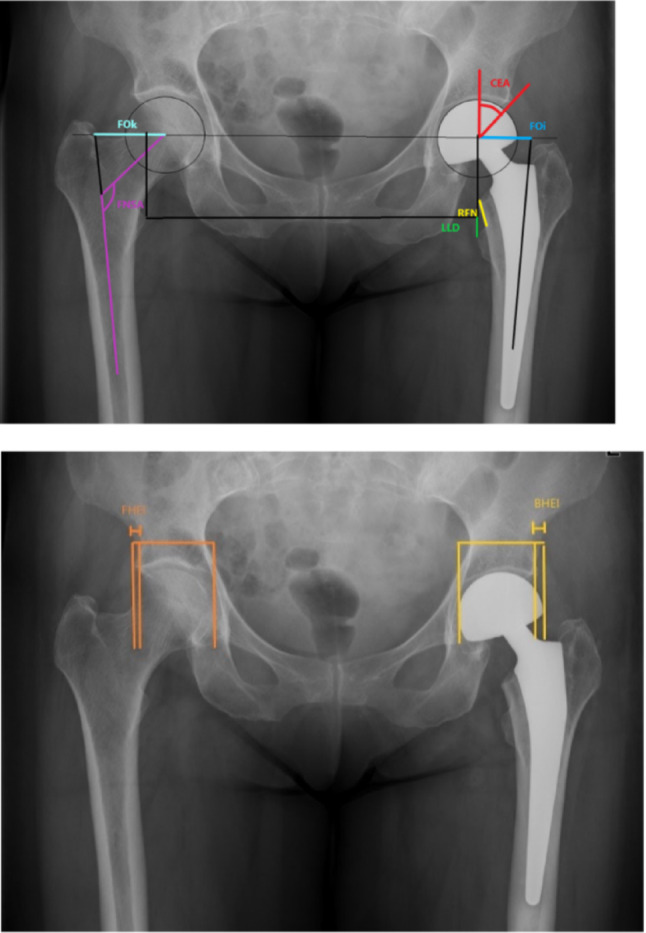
Measurement of radiographic parameters. Abbreviations: BHEI = bipolar head extrusion index, FHEI = femoral head extrusion index
